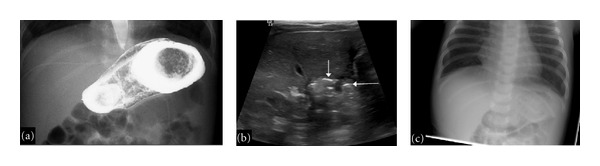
Radiographic findings of a neonatal gastric lactobezoar. (a) Upper GI demonstrating a large filling defect outlining the lumen of the stomach. (b) Sagittal ultrasound of the abdomen showing highly echogenic intrabezoaric air trapping (arrows) within a low echogenic lactobezoar. (c) AP chest X-ray with suggestive findings of a GLB. Intraluminal air outlines an opaque intragastric mass.
